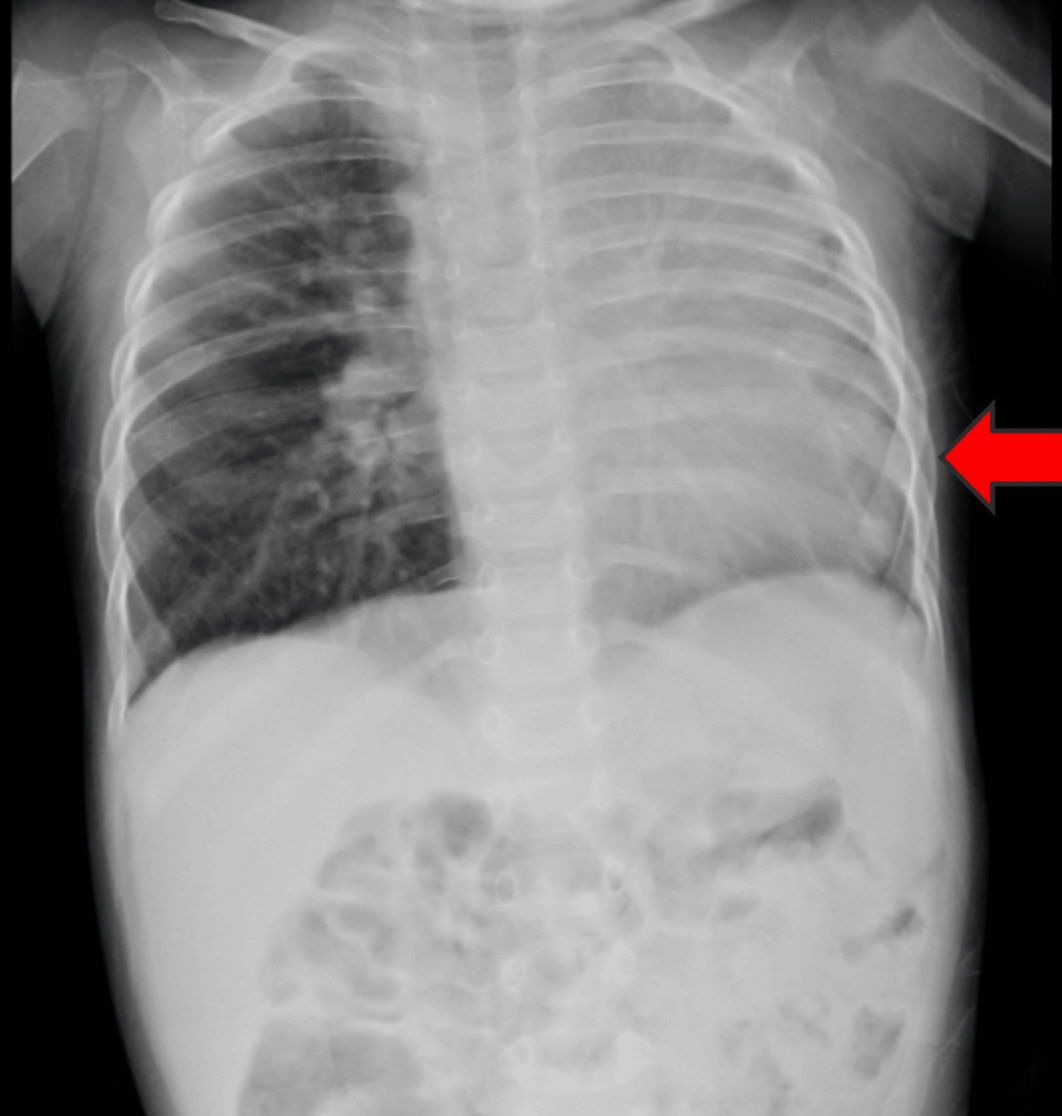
Chest X-ray revealing hypoplasia of the left lung (red arrow)
